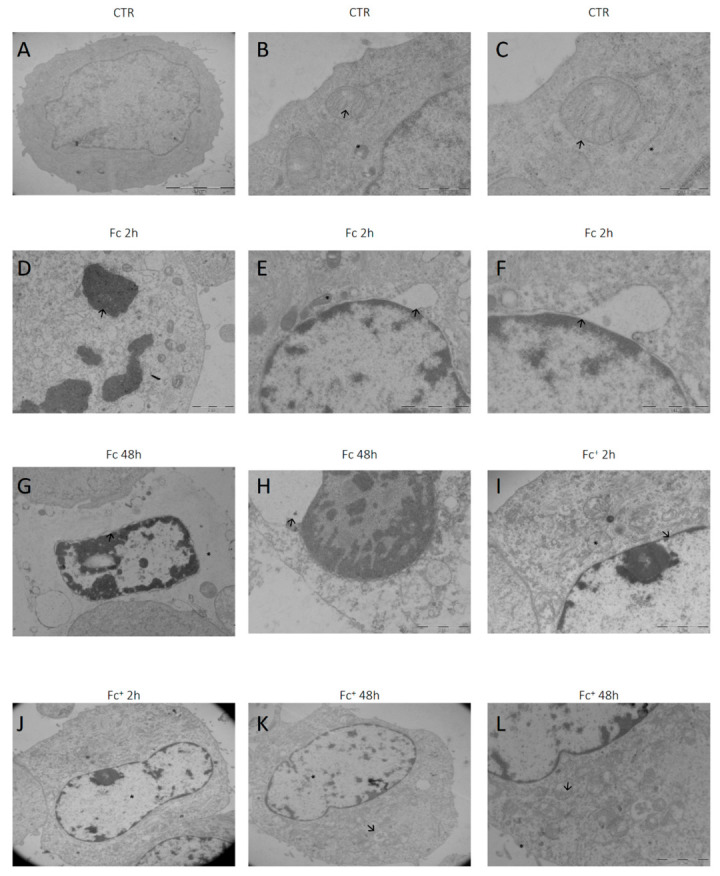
Ultrastructural analysis by TEM. (A–C) MCF-7 cells under control (CTR) conditions. (B,C) Healthy mitochondria with well-organized cristae, indicated by arrows. (D,F) Cells after 2 h of continuous treatment with Fc 200 μM. (D) Apoptotic and (E) necroptotic cells with altered mitochondria (indicated by an asterisk in E) and a detached perinuclear space, highlighted by arrows in (E,F). (G,H) Cells exposed for 48 h to Fc 200 μM. Advanced necroptotic phase showing an electron-lucent cytoplasm (indicated by an asterisk in G), a more condensed chromatin, and an extreme enlargement of the perinuclear space (arrows). (I,J) Cells treated for 2 h with Fc^+^ 200 μM. No enlargement of the perinuclear space was observed (arrow in I). Examples of altered mitochondria with impaired cristae structure (highlighted by asterisk in I) and ferroptotic cell with a decondensed chromatin (pointed by asterisk in J). (K,L) Cells after 48 h of CT with Fc^+^ 200 μM. Examples of late ferroptosis (K,L) characterized by decondensed chromatin (asterisk in K) and severely impaired mitochondria (highlighted by arrows in K,L).
